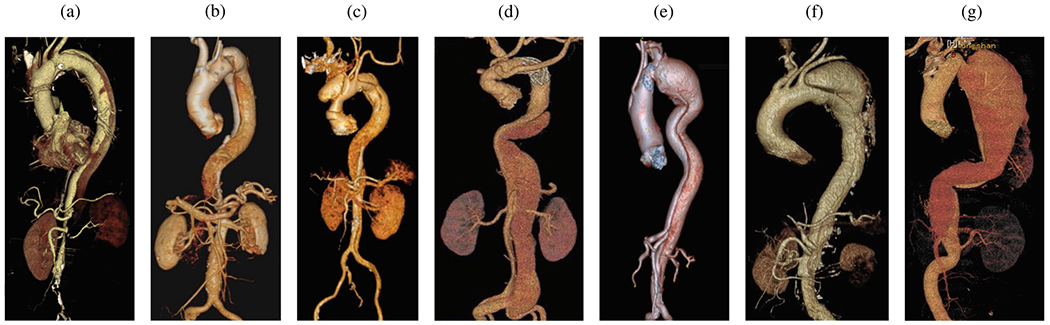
(a) to (g) CTA images demonstrate various remodeled anatomies of aortic dissection patients and showcase dilatation and growth of the aorta, including altered elongation, tortuosity, and angulation. Figure (a) is adapted from Chan et al. [294] with permission from Elsevier, while (b) and (c) are from Lopes et al. [295] (licensed under CC-BY-NC-ND 4.0), (d) is from Dai et al. [296] (licensed under CC-BY-NC 4.0), (e) is from Sultan et al. [297] (licensed under CC-BY 4.0), (f) is from Anwar and Hamady [298] (licensed under CC-BY 4.0), and (g) is from Ma et al. [299] with permission from Elsevier.
